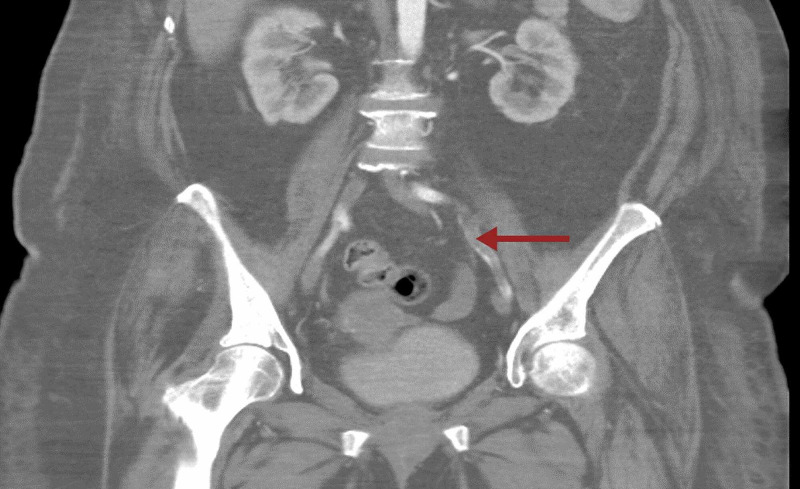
CT angiogram of the abdomen showing long segment thrombus in the external iliac artery.
